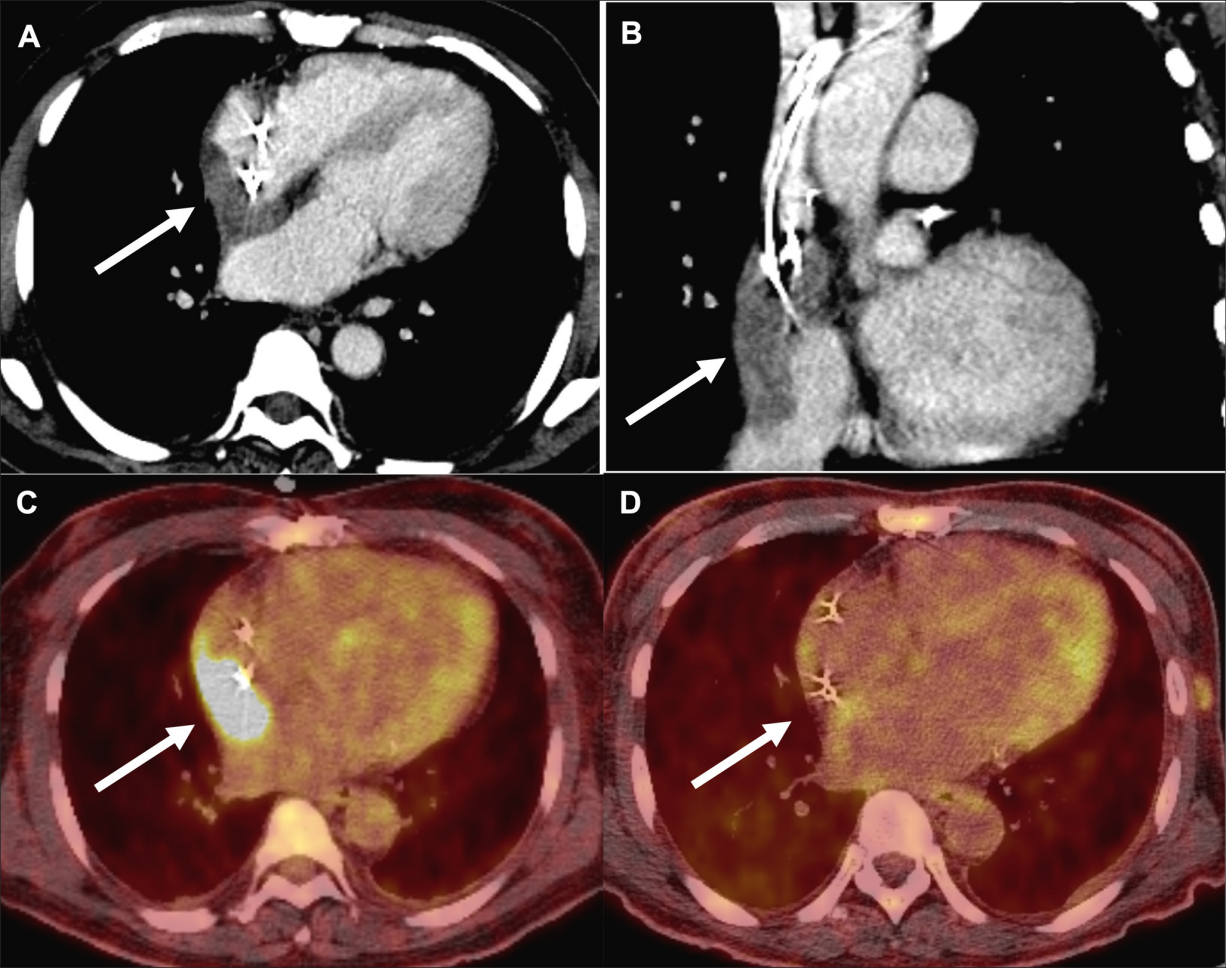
(A) Mass-like right atrial filling defect (arrow) demonstrated on transverse contrast computed tomography (CT). (B) Right atrial mass (arrow) redemonstrated on sagittal contrast CT encasing pacemaker leads. (C) Prechemotherapy positron emission tomography with computed tomography (PET-CT) demonstrating avid uptake of a right atrial mass (arrow) encasing pacemaker leads in transverse view. (D) PET-CT demonstrating complete metabolic response (arrow) post-treatment in transverse view.
